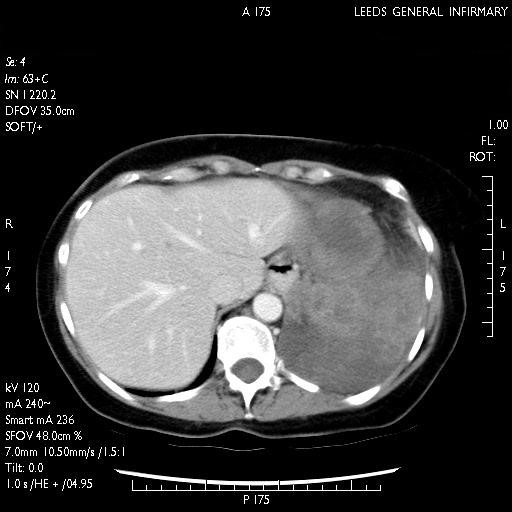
CT scan depicting a well circumscribed tumor measuring 10 cm antero-posteriorly, 14 cm transversely and 9 cm cranio-caudally enveloping the descending aorta and extending into the posterior mediastinum.
